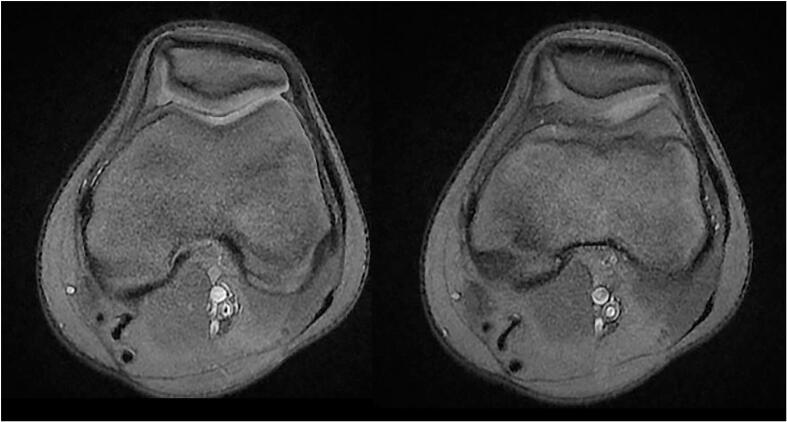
MRI of the left knee showing no plica (1.5 years after arthroscopic resection).
